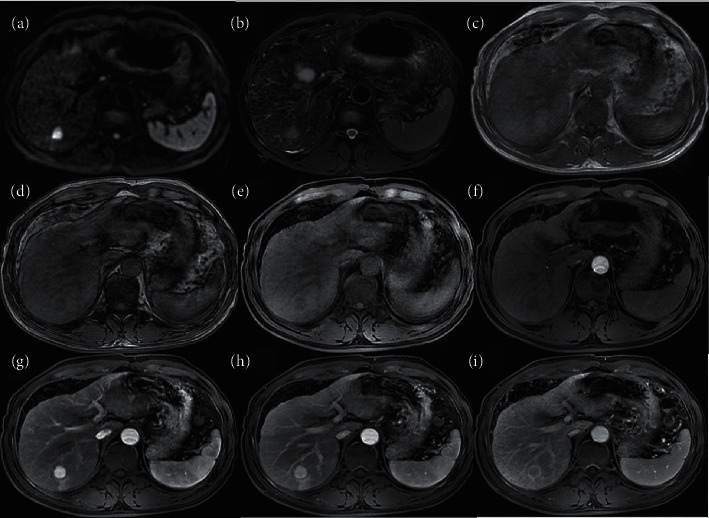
MR images in a 46-year-old HBV-positive man with iCCA confirmed by surgical resection, and this case was miscategorised as LR-5. (a) DWI image; (b) T2WI; (c) T1WI image in-phase; (d) T1WI image out-phase; and (e) 3D fat-suppressed T1WI precontrast and in the (f) early arterial, (g) later arterial phase, (h) portal phase, and (i) delayed phases. The tumor measures 17 × 17 mm and shows APHE, nonperipheral “washout” appearance and capsule.
